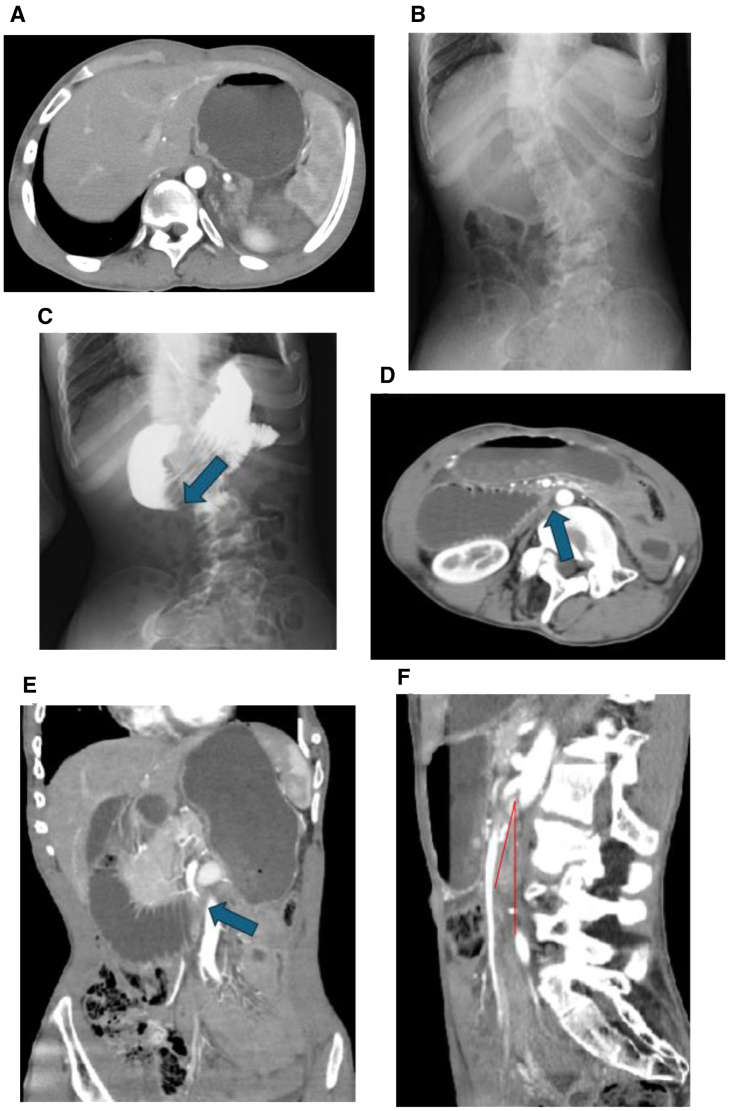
Clinical and radiological findings of the patient. (A) Abdominal contrast-enhanced CT showed increased fat stranding around the pancreatic body and tail. (B) Standing plain abdominal X-ray revealed dilation of the stomach and duodenum. (C) During an upper gastrointestinal contrast study performed in the standing position, the passage of contrast medium from the stomach into the duodenum was smooth; however, a stricture was observed in the horizontal portion of the duodenum, and the contrast medium did not flow beyond that point. (D, E) Axial and coronal views of contrast-enhanced abdominal CT demonstrated dilation of the stomach and the horizontal duodenum compressed between the superior mesenteric artery and the aorta (arrow, beak sign). (F) In the sagittal view, the aortomesenteric angle was narrowed to 18°, compared with the normal angle of approximately ≥22°. CT = computed tomography.
